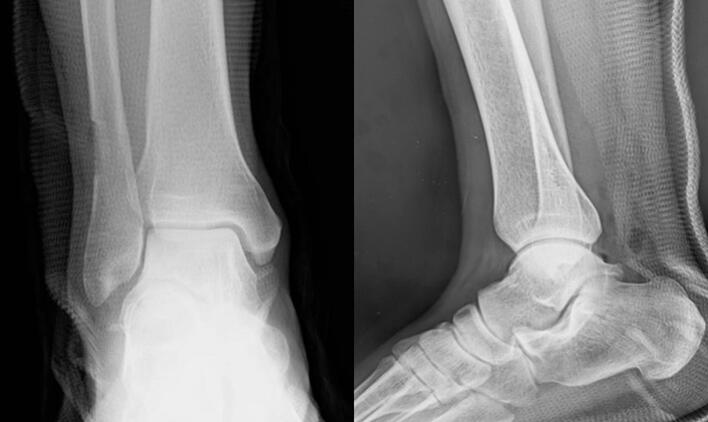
Radiograph taken immediately after surgery.
